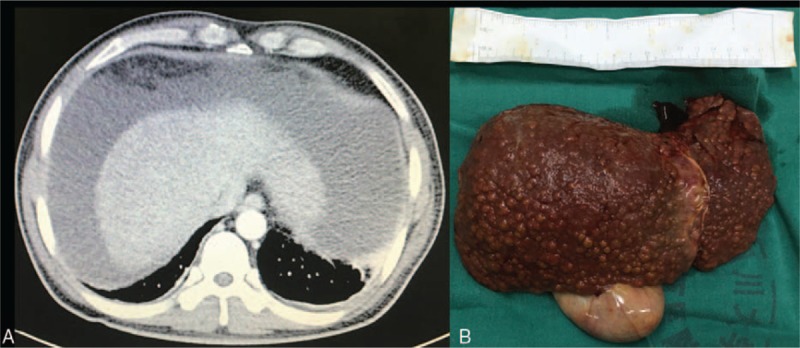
Preoperative contrast-enhanced abdominal computed tomography scan and the resected liver of recipient. (A) Computed tomography enhanced scan of the upper abdomen showed massive ascites and atrophic liver, (B) the gross multinodular cirrhosis appearance of the explanted liver.
